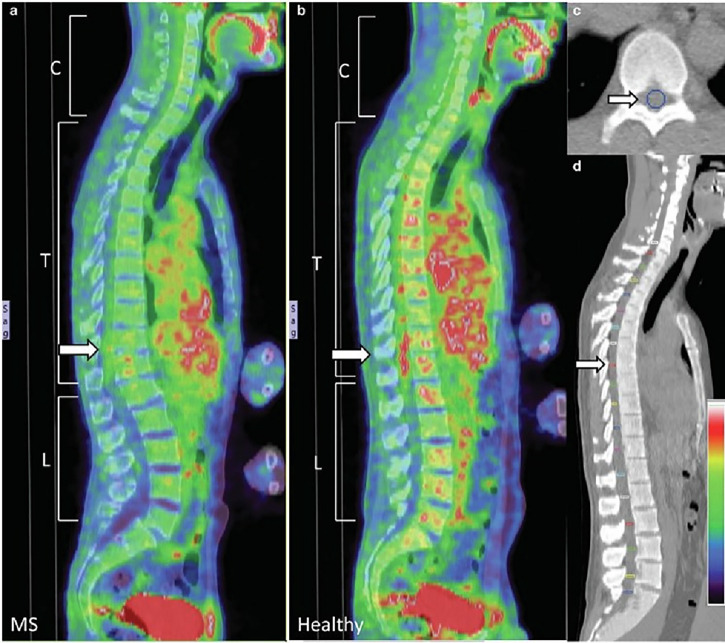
Representative Spinal Cord Glucose Uptake in an MS Patient and a Healthy Control. PET/CT images for a patient with MS (a) and a healthy participant (b). Increased [18 F]-FDG uptake is indicated by red and lower intensity indicated by blue. Note the difference between T11 and T12 and the reduced (18 F)-FDG uptake in this area in the patient with MS. c A transverse image from the CT scan shows a region of interest (ROI) drawn at the mid-vertebral body level. d A sagittal view of the CT image demonstrating ROI drawn throughout the spinal cord at the mid-vertebral body level [6].
